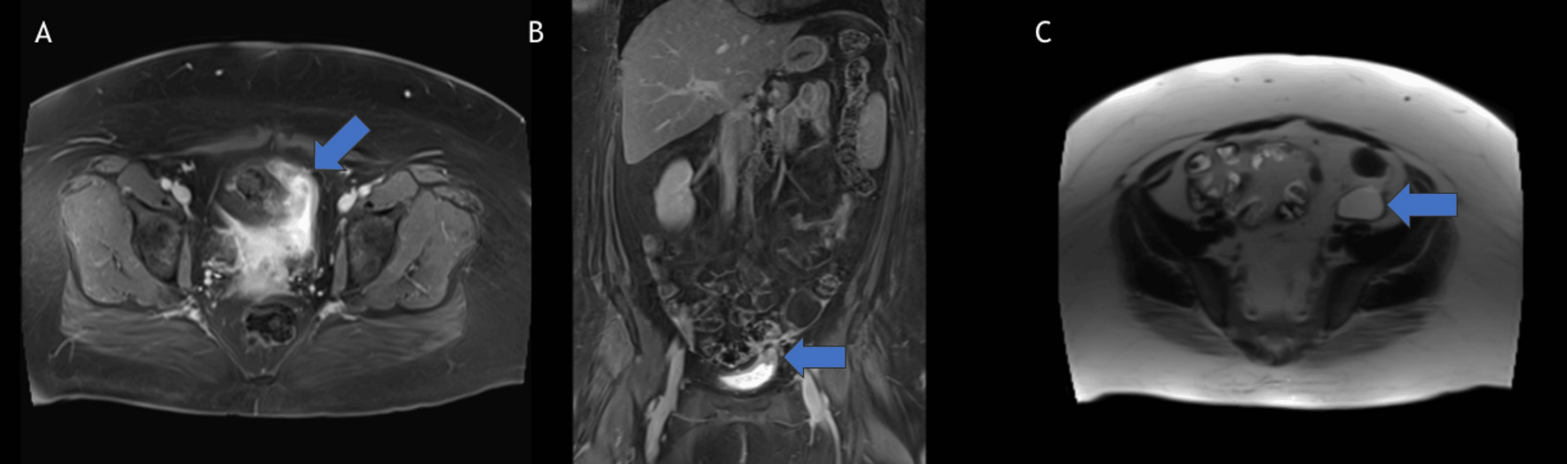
Magnetic resonance (MR) urogram February 2025 (4A) Transverse view with T1 contrast enhanced fat saturated Magnetic Resonance Imaging (MRI) demonstrating focal contact between a sigmoid diverticulum and the left bladder base, with some surrounding inflammatory change suggestive for colovesicular fistula. (4B) Coronal view with T1 contrast enhanced fat saturated Magnetic Resonance Imaging (MRI) demonstrating focal contact between a sigmoid diverticulum and the left bladder base, with some surrounding inflammatory change suggestive for colovesicular fistula. (4C) Transverse view with T2 weighted MRI demonstrating hyperintense contents of previously seen left adnexal lesion without any septations or nodularity, representing fluid and suggestive of a simple left adnexal cyst.
